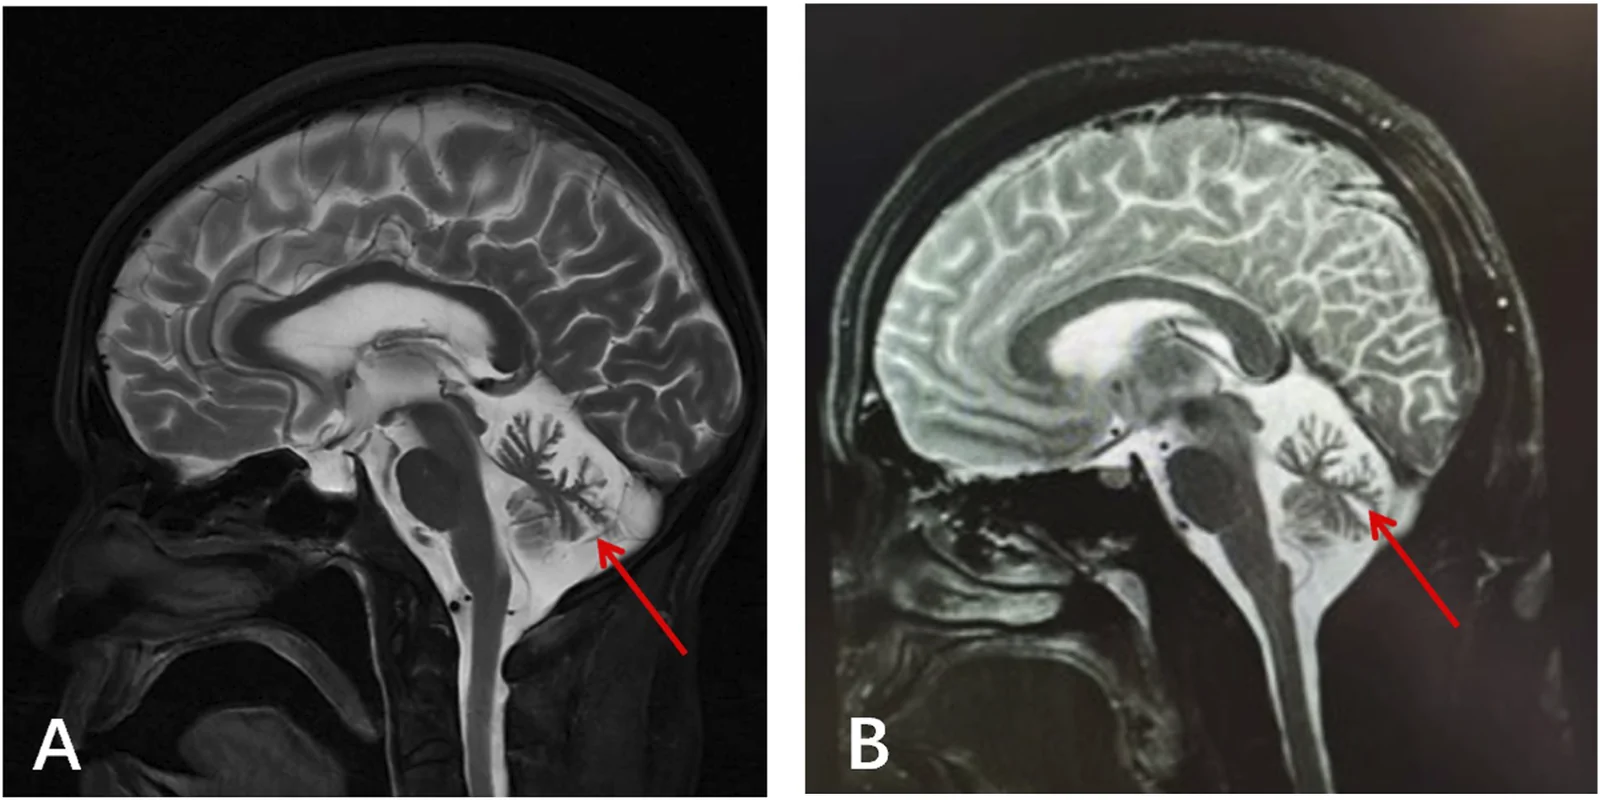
Head MRI of the patient and her son. Note: (A) In December 2024, an MRI scan of the patient indicated cerebellar atrophy. (B) An MRI of her son showed that the bilateral cerebral sulci were widened, and the bilateral cerebellar crus were narrowed.
